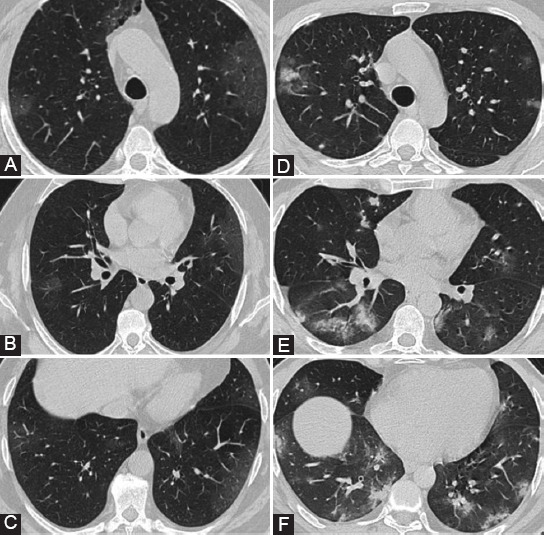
(A-C) Axial computed tomography (CT) images of a 39-year-old man with coronavirus disease 2019 (COVID-19) pneumonia showed bilateral ground-glass opacities with dominantly peripheral distribution. All lung lobes were involved and the Zonal CT Visual Score was 14. Patient was discharged from the hospital without intensive care unit (ICU) admission. (D-F) 67-year-old man with laboratory confirmed COVID-19 pneumonia. Axial chest CT images demonstrated bilateral consolidation, ground-glass opacities, halo sign, and vascular enlargement. The lesions involved in five lung lobes and the Zonal CT Visual Score was 16. He recovered without ICU admission.
